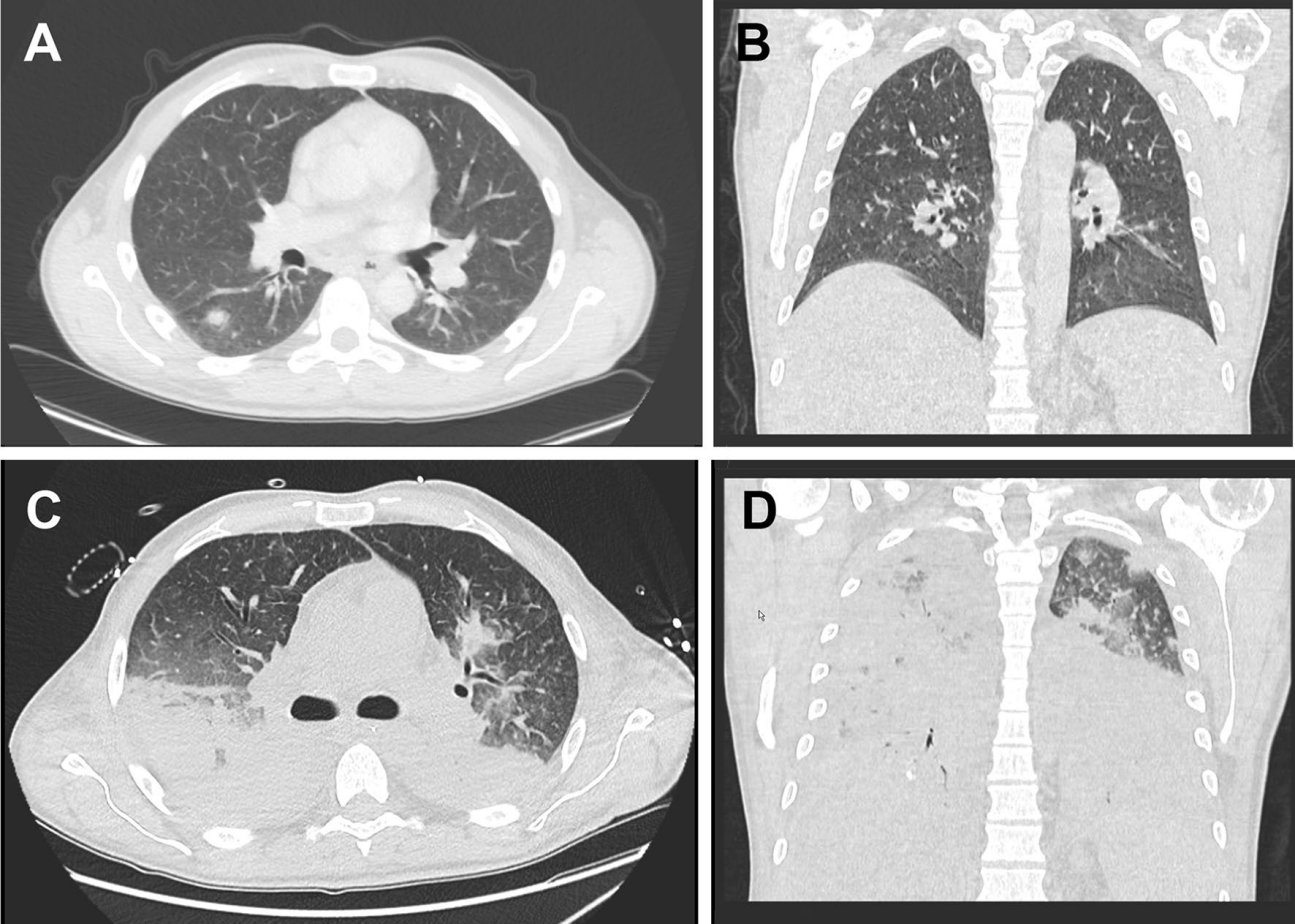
CT images show pulmonary infiltrates initially presumed to be due to fungal infection or leukaemic manifestations at the time of admission (A/B) and progressive diffuse opacities due the development of severe ARDS 5 days later (C/D)
